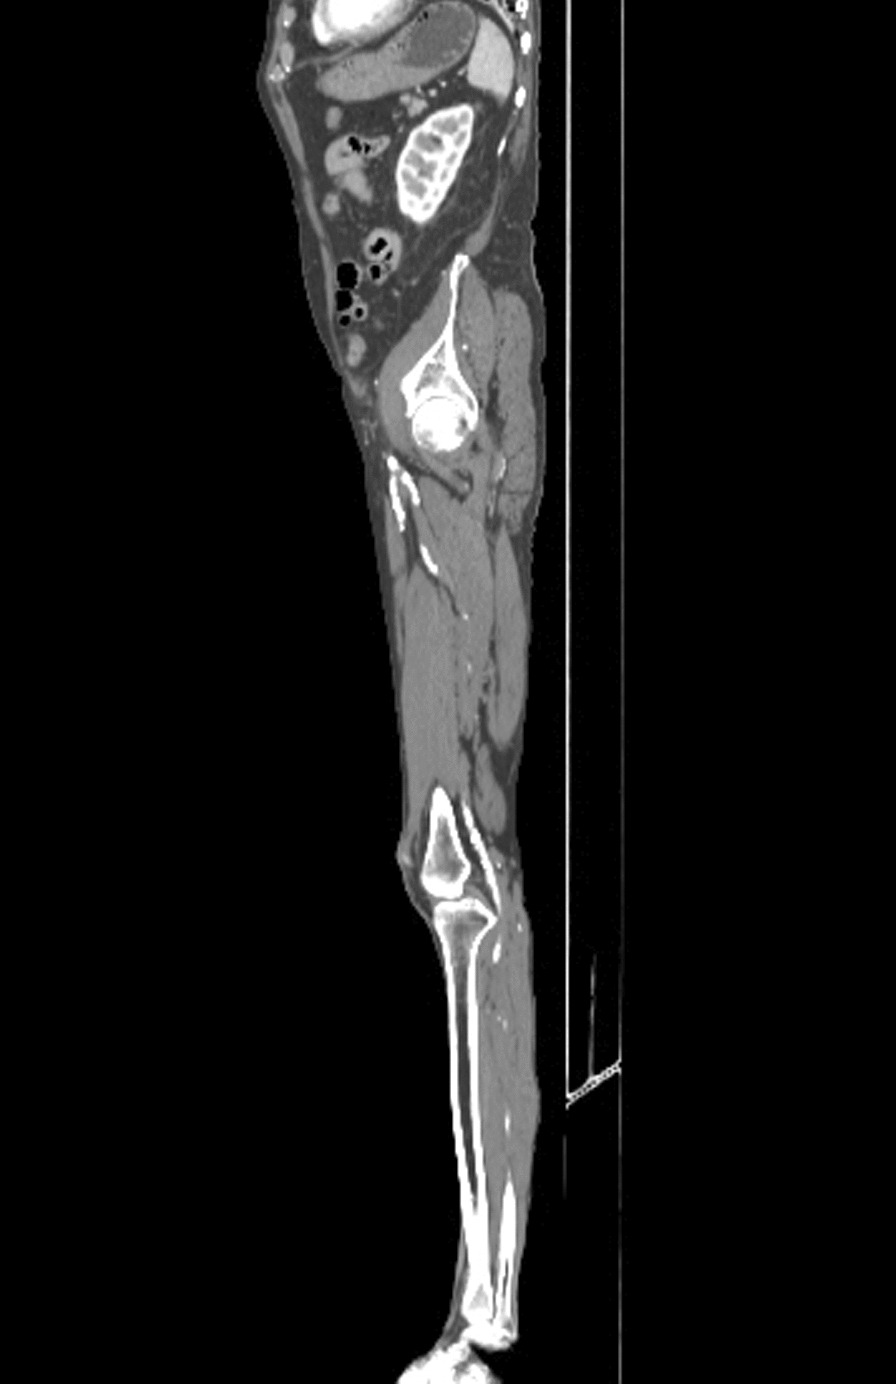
CT angiography showing the thrombus affecting the superficial and deep femoral arteries on the left side
